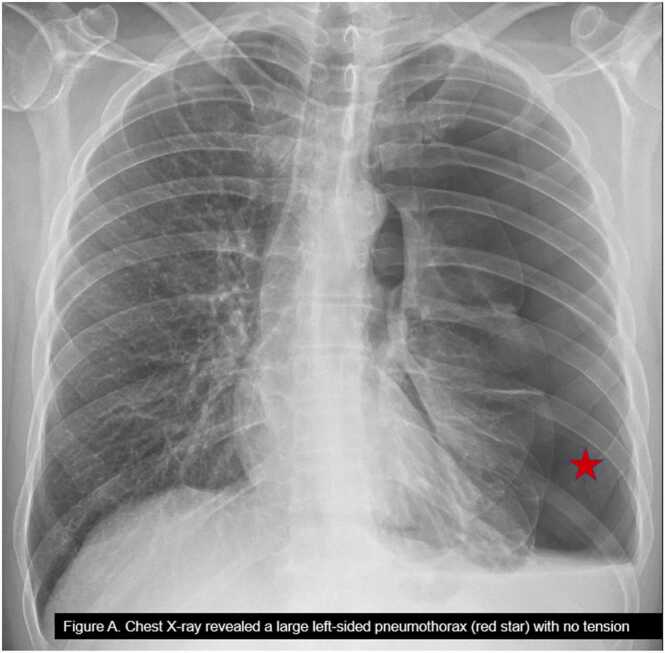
Chest X-ray revealed a large left-sided pneumothorax (red star) with no tension.
